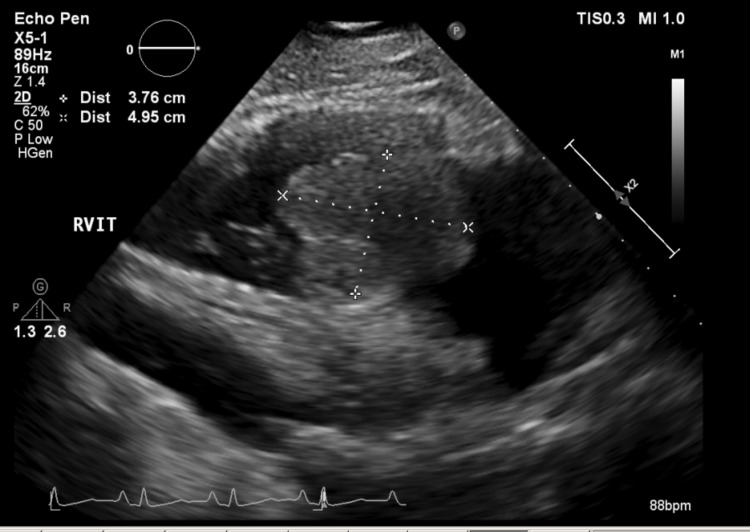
Transthoracic echocardiogram with RV inflow window showing a large mass measuring 3.7 × 4.9 cm. RV: right ventricular
